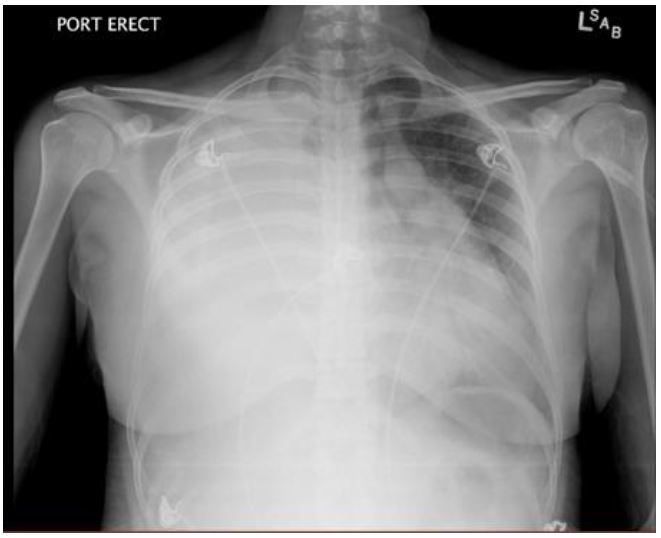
Portable anteroposterior radiograph of the chest reveals complete atelectasis of the right lung (white arrow), adjacent right-sided pleural effusion (arrowhead) and leftward mediastinal shift (black arrow) consistent with tension hydrothorax.
